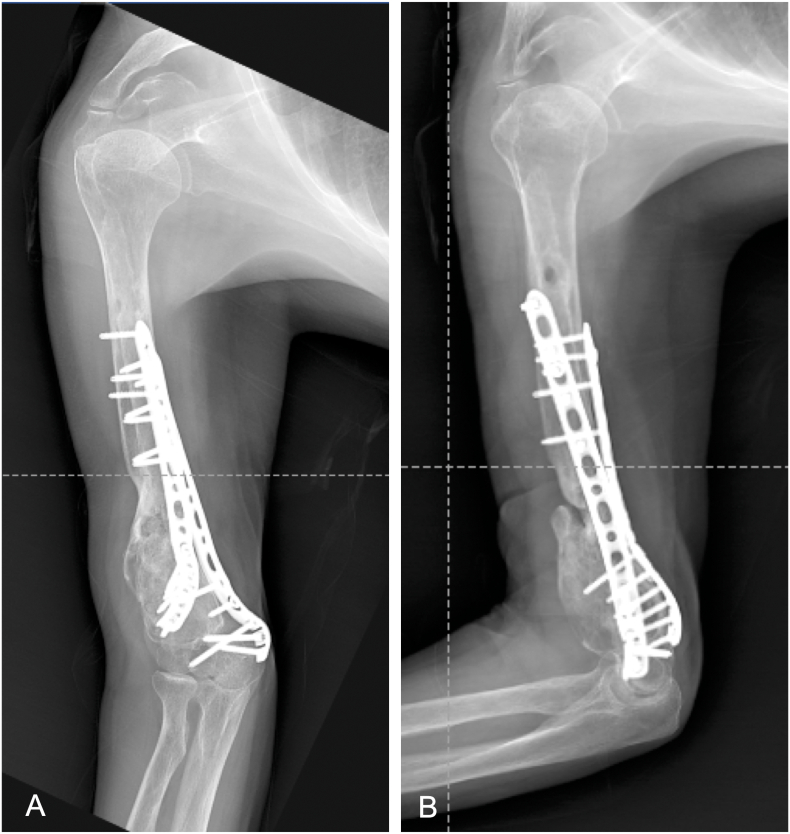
X-ray findings one year after the surgery. A) Anterior posterior view of the humerus. B) Lateral view of the humerus.
